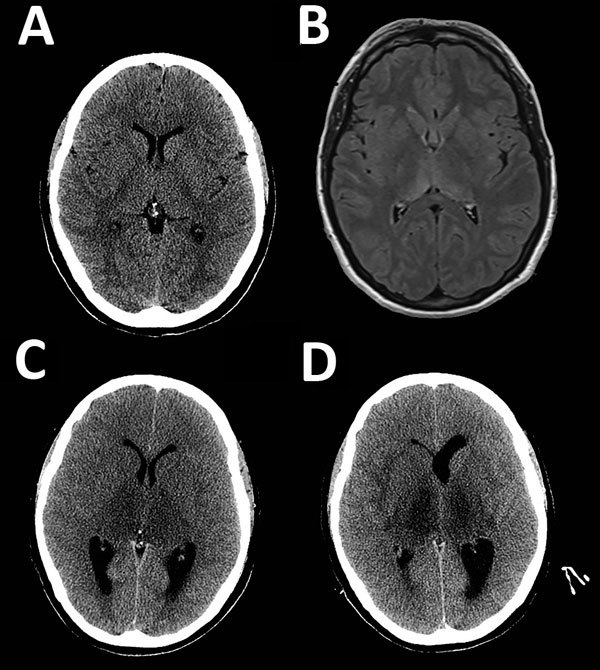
Neuroimaging during course of illness for a patient with a fatal infection of Murray Valley encephalitis virus imported from Australia to Canada, 2011. Each image corresponds to an axial cross-section through the thalamus and basal ganglia. A) Computed tomography (CT) at day 3. B) Magnetic resonance imaging (T2 flipped attenuation inversion recovery sequence) at day 3 showing abnormalities in the posterior thalami and splenium of the corpus callosum. C) CT when a fixed, dilated, right pupil (day 8) developed in the patient showing marked thalamic hypo-density and obstructive hydrocephalus. D) CT before death (day 10) showing necrosis of both thalami and a dilated left lateral ventricle.
